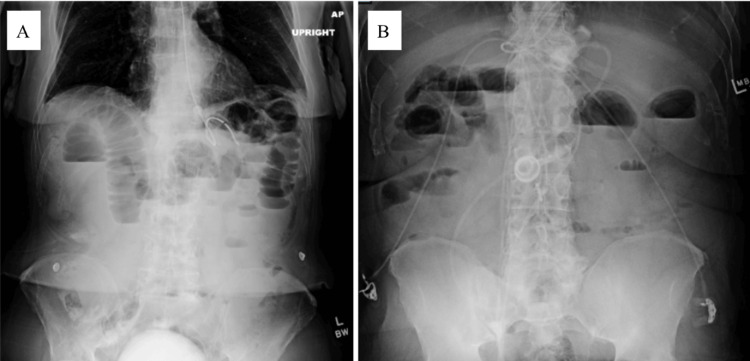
Representative AXRs of control patients (A) AXR demonstrating SBO with dilated loops of small bowel; no oral contrast is visible. (B) AXR demonstrating SBO with prominent air-fluid levels; no oral contrast is visible. AXR, abdominal radiograph; SBO, small bowel obstruction
